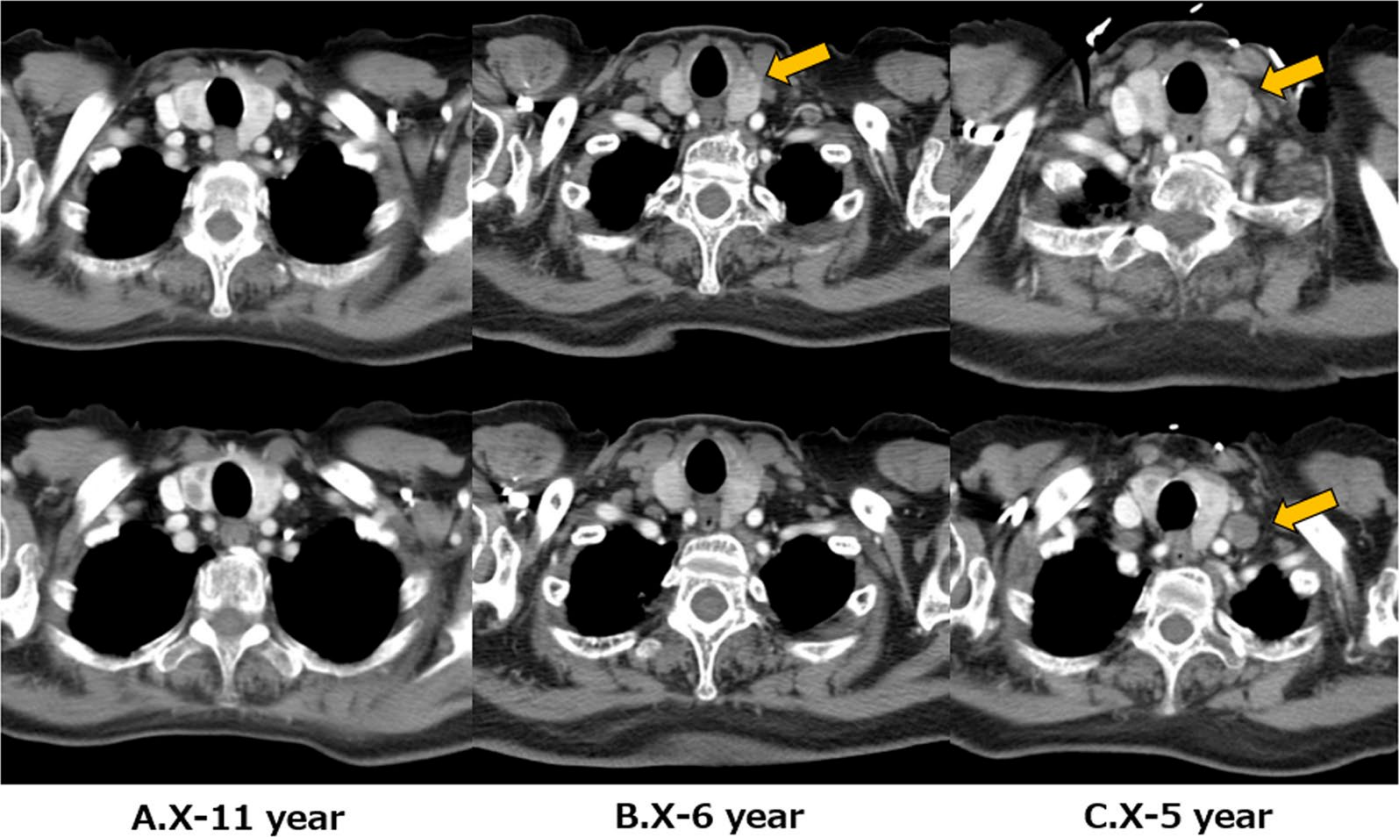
Review of previous CT findings. A Multiple thyroid nodules are seen within the thyroid gland. B Appearance of primary papillary thyroid carcinoma. C Appearance of left VI lymph node metastasis
